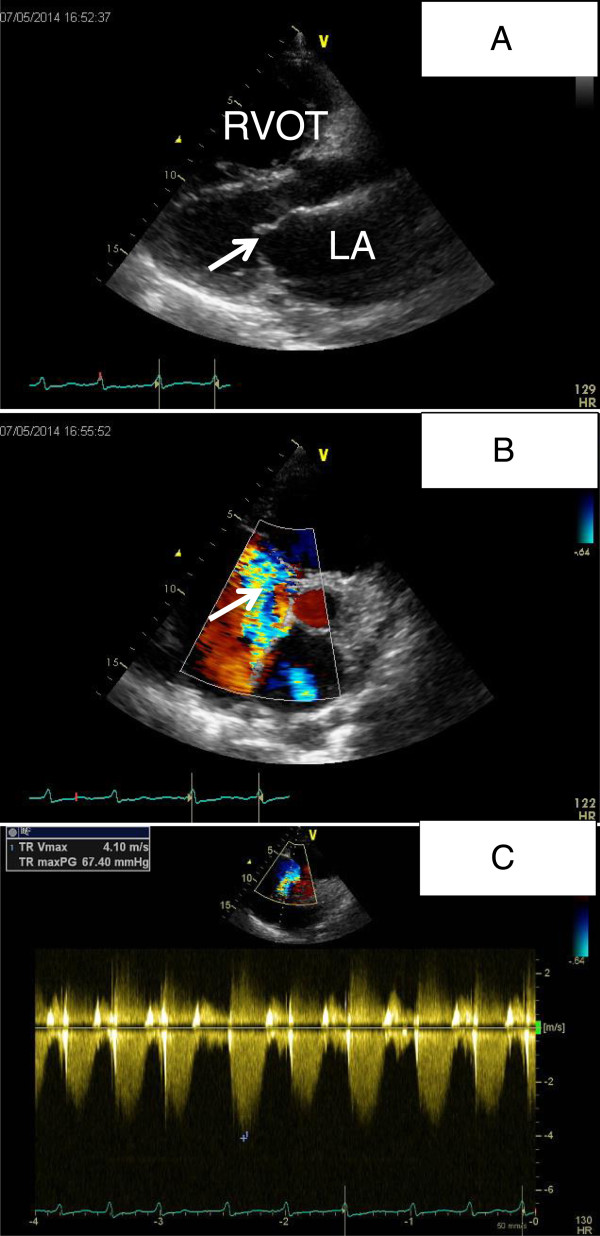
TTE revealed PAH with right-sided chamber enlargement. A: Dilatation of the RVOT(right ventricular outflow tract) was shown. B: Severe tricuspid regurgitation was shown in the parasternal short axis view (white arrow) with dilatation of RV and RA. C: The pressure gradient between the RV and RA was around 67 mmHg by measuring the tricuspid regurgitation Doppler jet.
